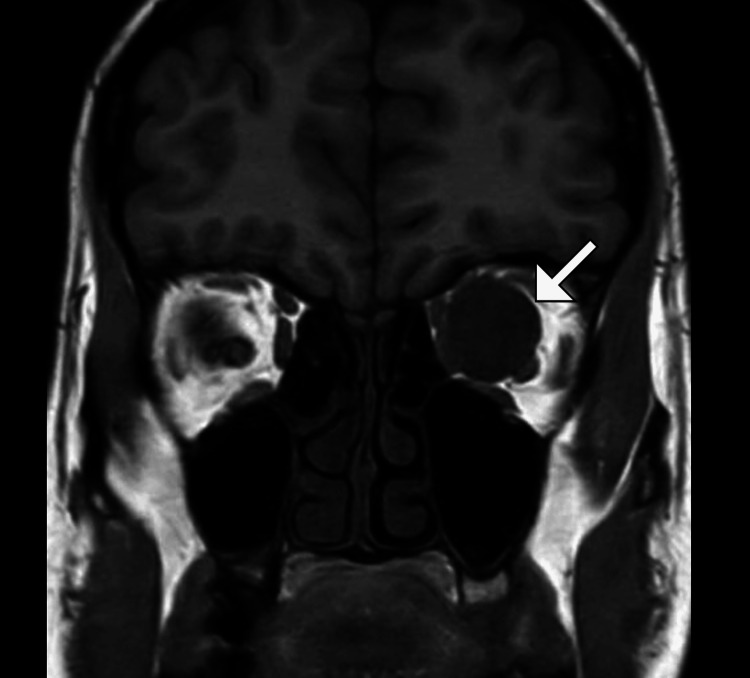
Coronal MRI T1-weighted pre-contrast image showing a well-circumscribed, hypointense, intraconal lesion (arrow) in the left orbit The lesion is located medial to the optic nerve, without evidence of invasion or compression of surrounding structures, consistent with a cavernous hemangioma.
